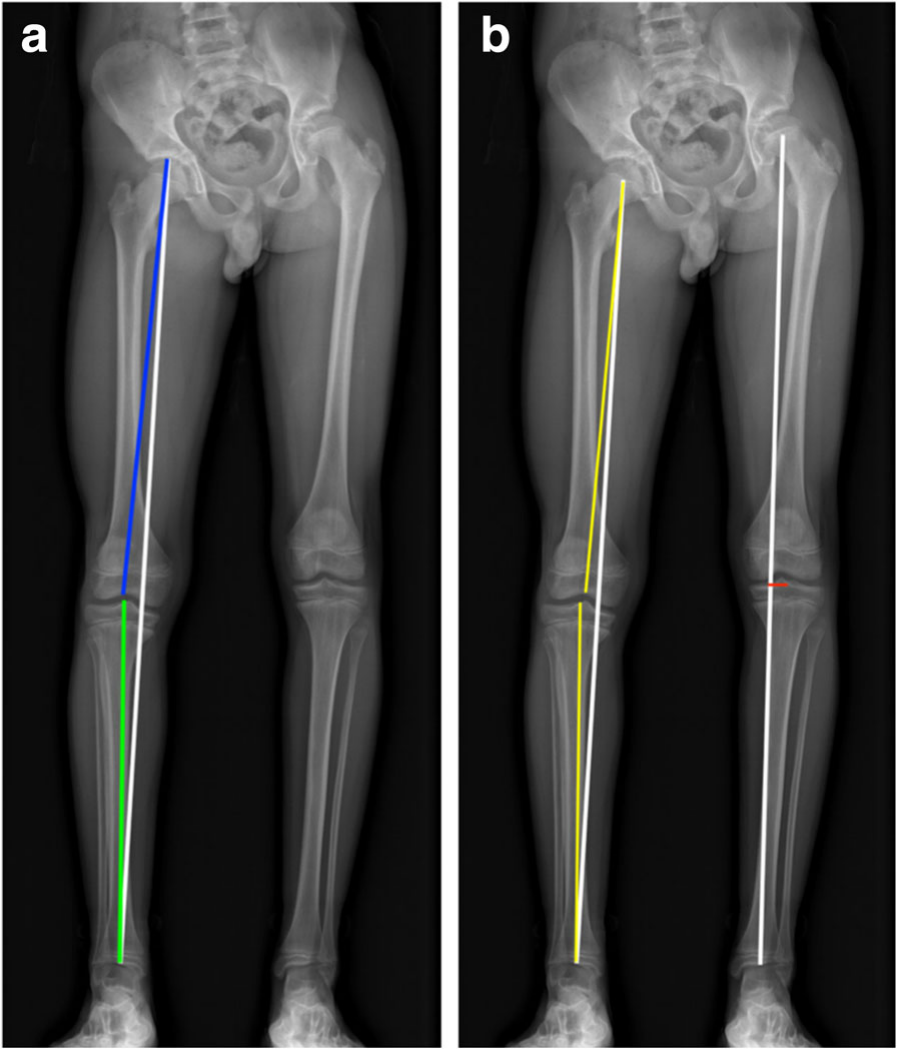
Measurements were performed on long-standing X-rays. a Limb length (white), femoral length (blue), tibial length (green). b Mechanical axis (white), mechanical axis deviation [MAD] (red), axial deviation (yellow)
